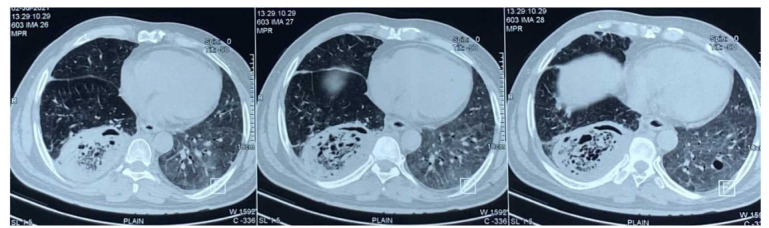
A 56-year-old male was admitted as a case of COVID-19-associated mucormycosis with respiratory symptoms. Computed tomography chest plain axial section shows evidence of a thick-walled cavity with central ground glass opacities and peripheral consolidation in the right lower lobe (bird’s nest sign) suggestive of pulmonary mucormycosis.
